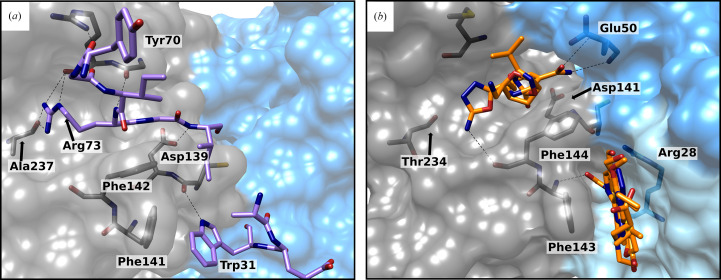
Comparison of the TrxR–Trx complex in *E. coli* with two fragment clusters at the Trx site in Msm-TrxR. (*a*) X-ray structure (PDB entry 1f6m; Lennon *et al.*, 2000 ▸) showing Trx interacting with the F_R_ conformation of *E. coli* TrxR (gray, NADPH-binding domain; blue, FAD-binding domain; purple, Trx). The Trx loop (Tyr70–Ile75; purple) interacts with a binding cleft in the NADPH-binding domain (gray) in the F_R_ conformation. (*b*) Fragments (orange) bound at the Trx interaction site can be separated into two clusters and interact with both domains and protomers (shades of gray, NADPH-binding domain; shades of blue, FAD-binding domain). Hydrogen bonds are shown as black dashed lines.
